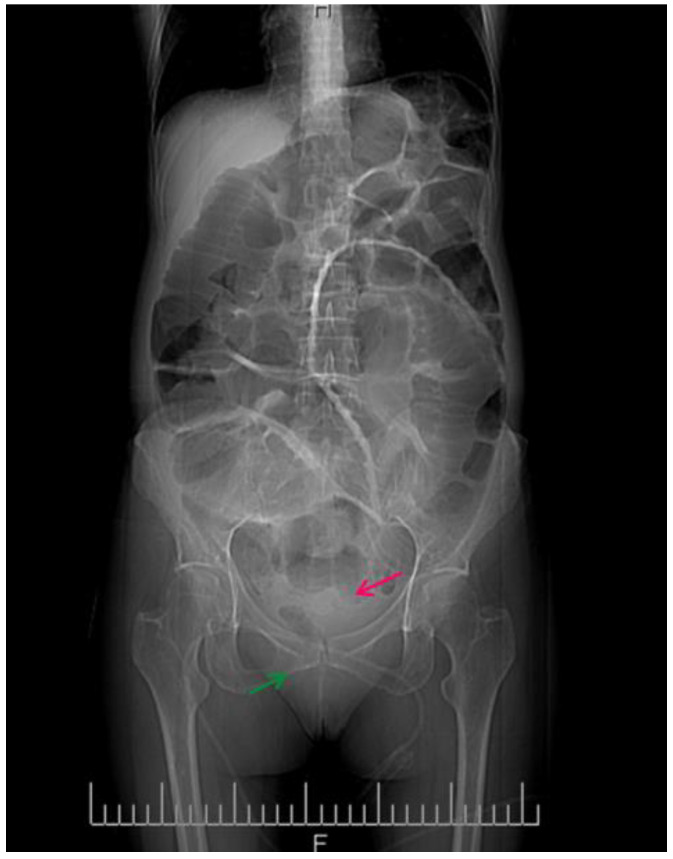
CT image of the intestinal volvulus—“whirlpool sign” (red arrow), air loops (green arrow).
